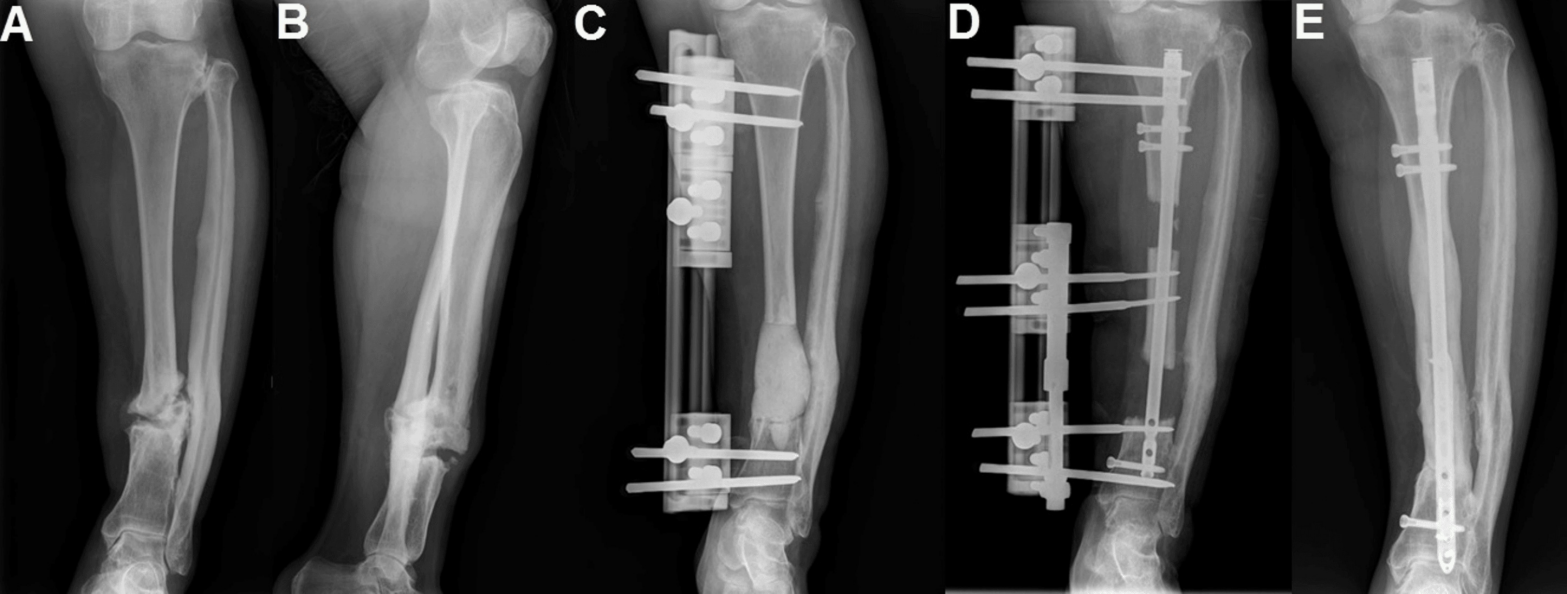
A 44-year-old female patient with a 12-year history of left tibia Cierny-Mader type IV B1 chronic osteomyelitis. (A, B) Anteroposterior and lateral radiographs of the patient.
